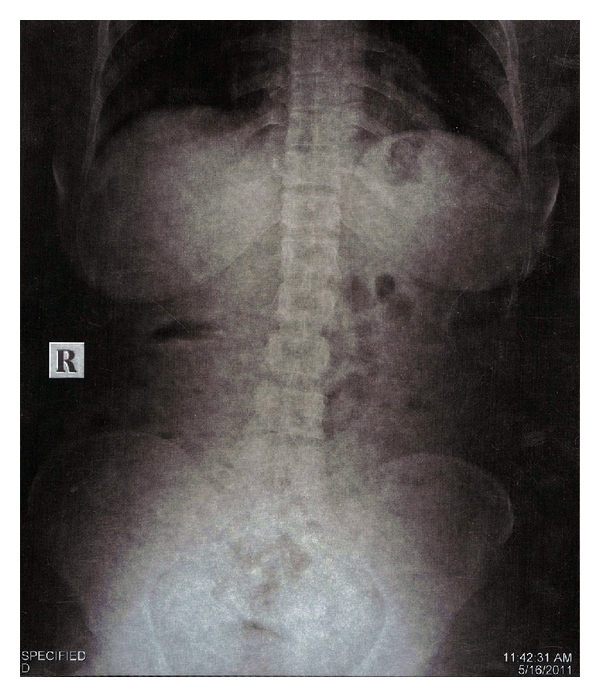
Abdominal radiograph after 3 days with no evidence of instrument.
